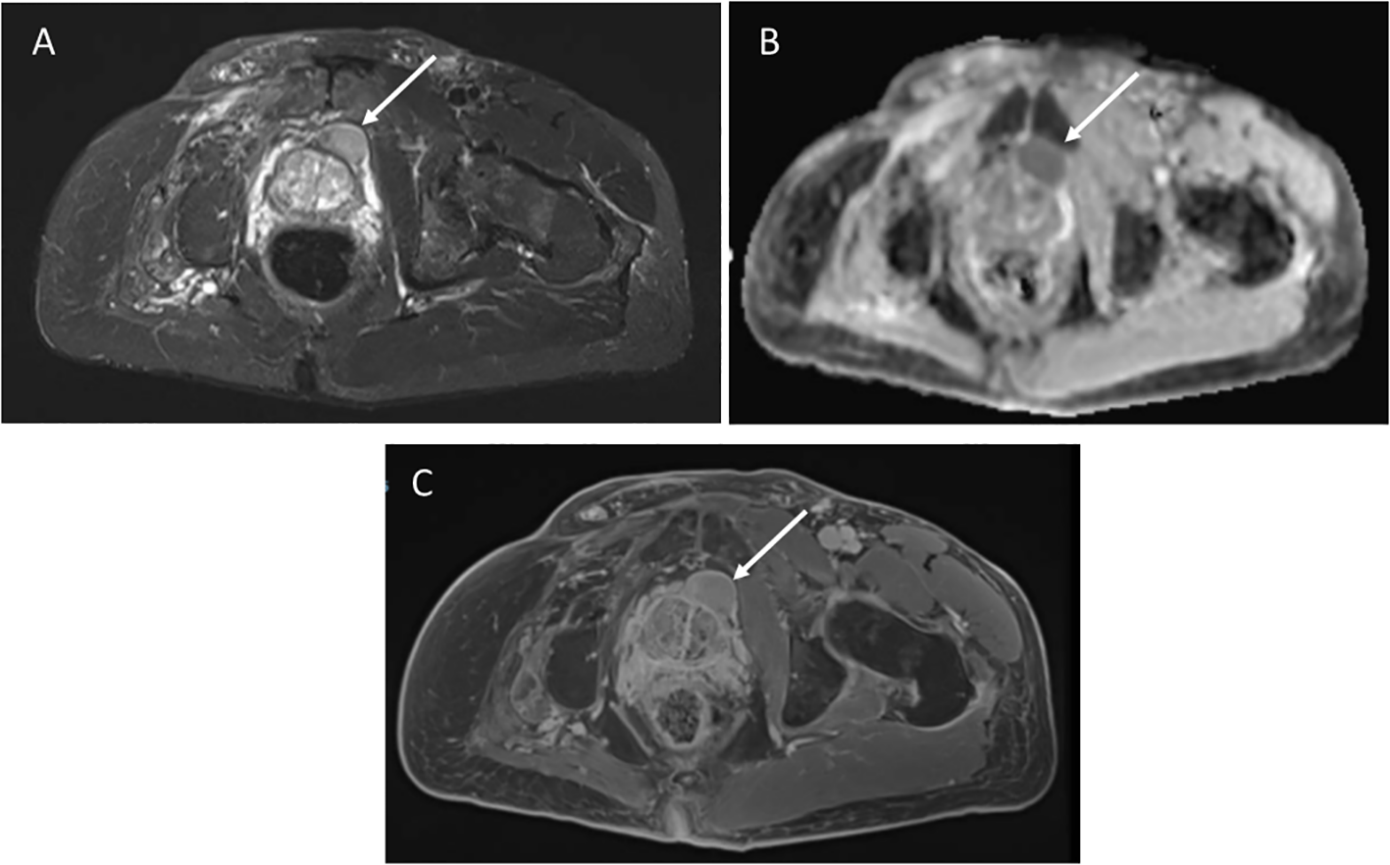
(A) Axial STIR image from whole body MRI study shows a mass anterior to the prostate gland, measuring 2.6 x1.5cm. (B) Apparent diffusion coefficient image shows restricted diffusion in the mass. (C) Axial post-contrast image demonstrates avid enhancement.
